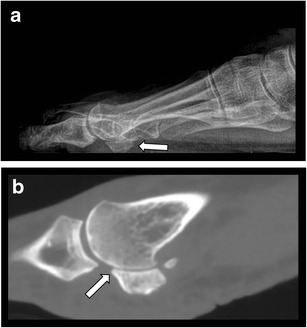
Sesamoid degenerative changes. a. CR lateral view of the foot in standing position with suspected degenerative changes between the sesamoids and the first metatarsal head. b. CBCT sagittal reformatted image shows osteophyte formation, narrowing of the joint space and subchondral sclerosis at the joint between the plantar aspect of the metatarsal head and the medial sesamoid (arrow)
